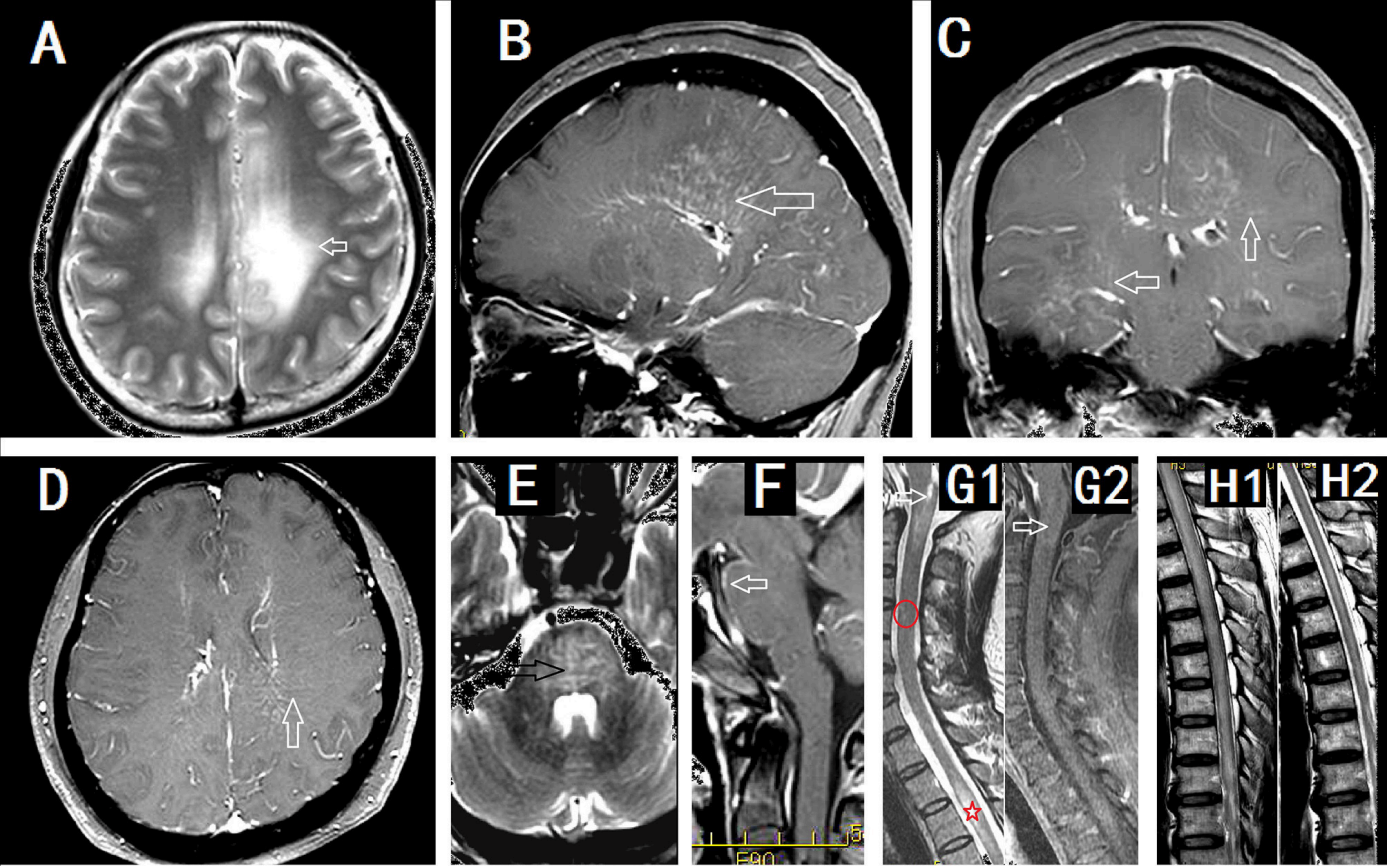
Imaging findings in patients with GFAP astrocytopathy. (A–D) were from a female meningoencephalitis patient. (A) MR images showing extensive abnormalities in the white matter around the ventricle (arrow). (B) Sagittal section showed linear perivascular radial gadolinium enhancement in the white matter perpendicular to the ventricle(arrow). (C,D) Coronal section (C) and cross section (D) showed vessel-like enhancement (arrows). (E) and (F) from a male meningoencephalitis patient showed pons abnormality (black arrow) and pia enhancement (white arrow). (G1) and (G2) were from a female with myelitis. (G1) Cervical lesion extended to the area postrema of medulla(arrow), sparse cervical abnormality(red round area) and thoracic LESCLs (star marker). (G2) Slightly enhancement in medulla (arrow). (H1) and (H2) were from a male meningoencephalomyelitis patient. Longitudinal extensive lesions in the whole spinal cord (H1) and soon recovery after the treatment (H2).
